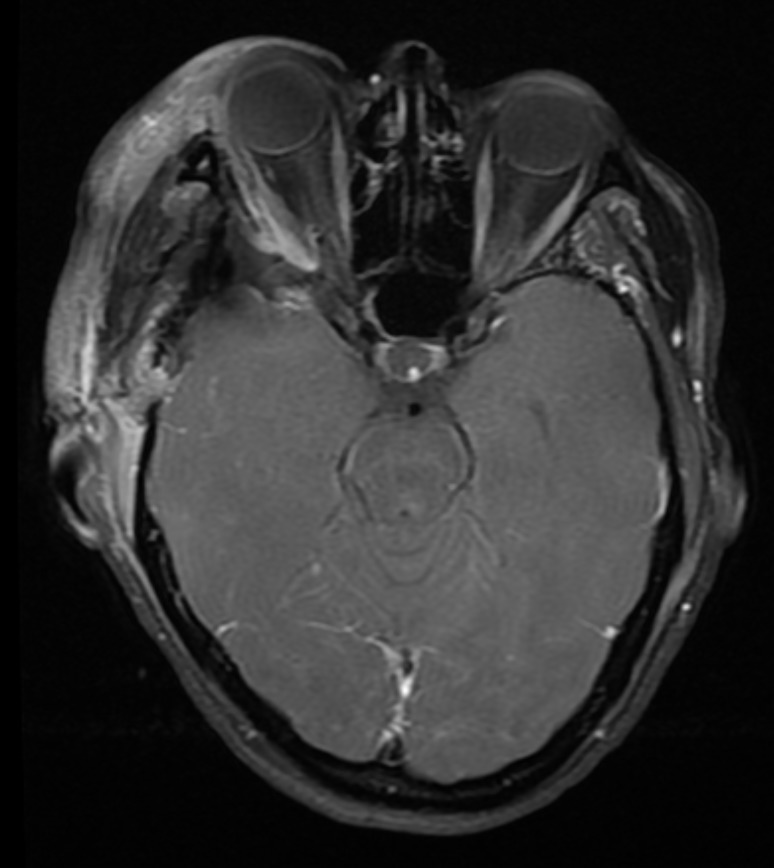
Postoperative MRI following right orbitozygomatic craniotomy for tumor resection Axial T1-weighted MRI with contrast showing near total resection.
